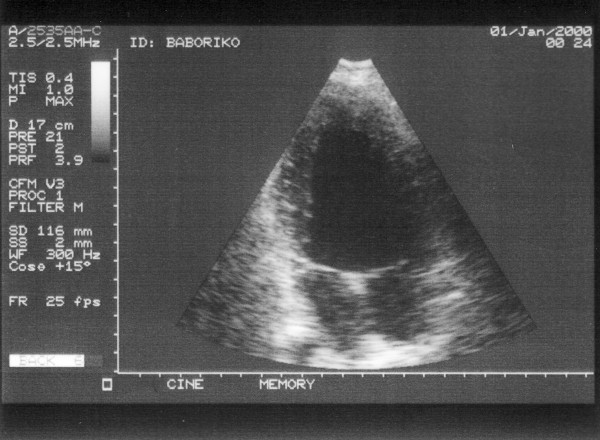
Echocardiogram from the terminal hospitalization. Apical view showing single ventricle with two atrioventricular valves.
